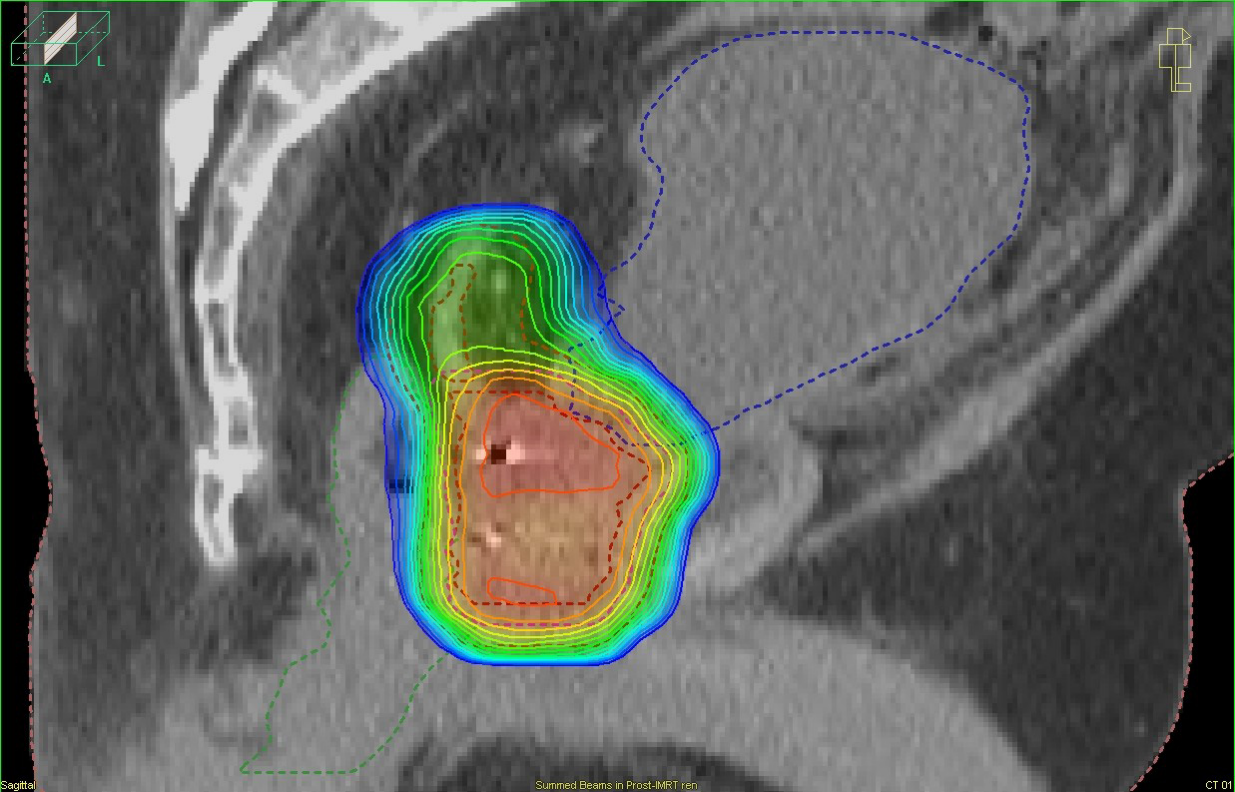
Intensity-modulated radiotherapy (IMRT) dose distribution for prostate cancer in a sagittal CT reconstruction.
